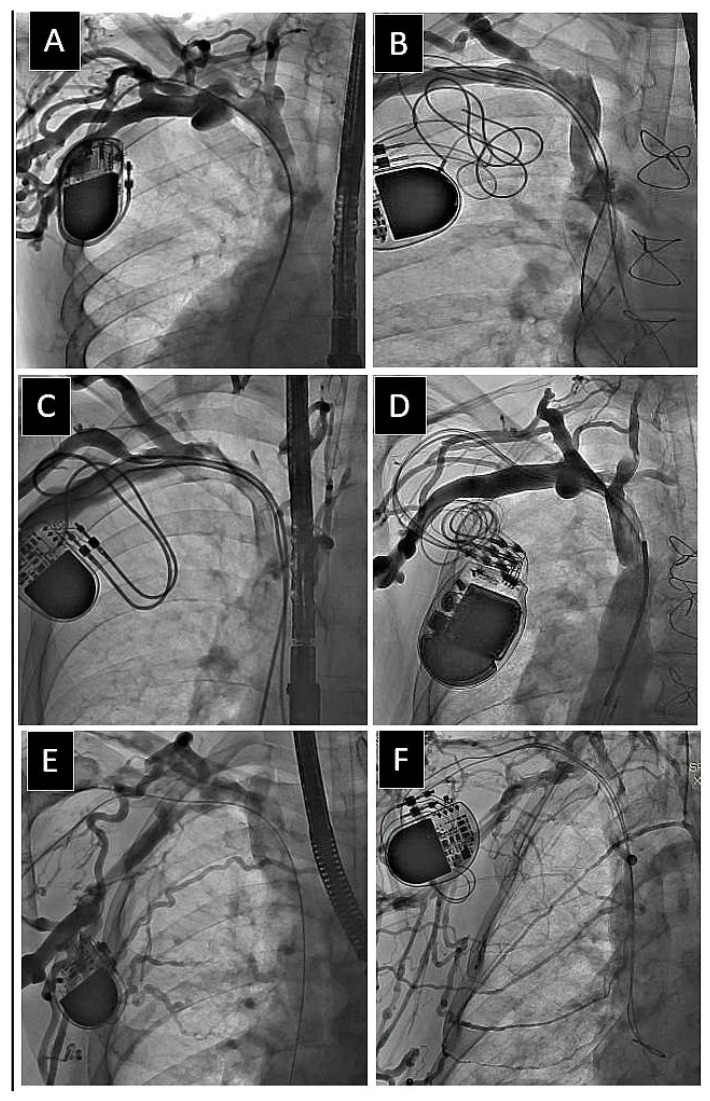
Six examples of lead-related obstruction of the major thoracic veins in patients with leads on the right side of the chest. A transesophageal echocardiographic probe can be seen. (A,B) Moderate stenosis isolated to one vein (right brachiocephalic vein). (C,D) Two veins with severe stenosis (right subclavian and right brachiocephalic veins). (E,F) Three veins affected. Severe stenosis of right subclavian and brachiocephalic veins and severe stenosis of superior vena cava. Collateral circulation through neck (A,D,F) and thoracic (E,F) veins.
